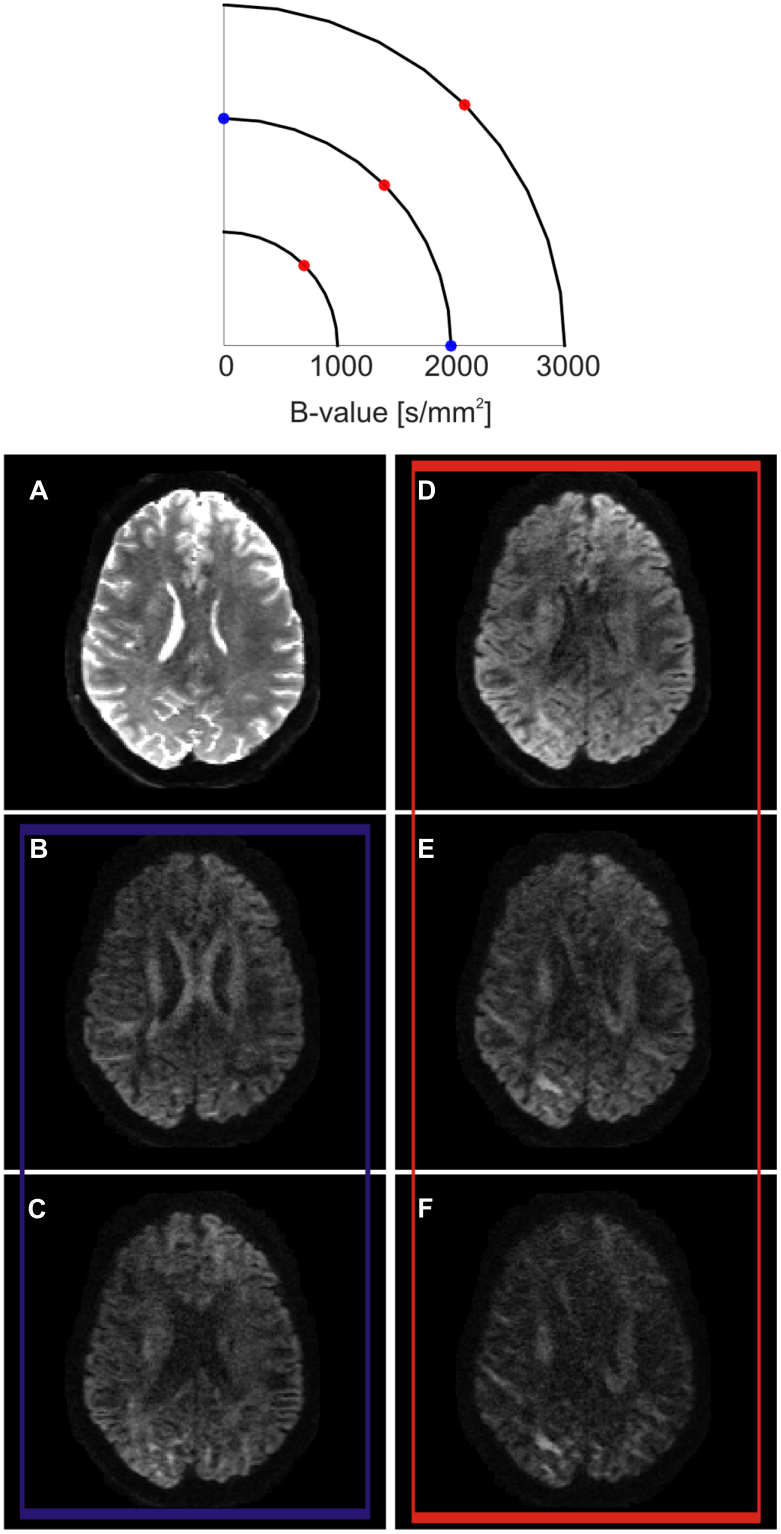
Q-space encoding example. Q-space is represented as a two dimensional space for simplification purposes (top). Three different shells at three different *b*-values are outlined. On each shell, several diffusion encoding directions (q-vectors) are sampled. Example axial slice obtained at different positions in q-space (bottom). (A) When the diffusion encoding gradients are switched off, the so-called T2-weighted b0 volume is obtained. (B,C) show the effect of sampling q-space at two different diffusion encoding orientations lying on the same b-shell. While SNR and amount of probed diffusion are kept constant, the contrast changes at different anatomical locations. When sampling along the left–right direction, the body of the corpus callosum show a higher signal drop (C) in respect to the sampling along the anterior-posterior direction (B). This reflects the fact that, in the body of the corpus callosum, axons are mainly oriented along the left–right orientation. (D–F) show the effects of sampling along the same diffusion encoding orientation while increasing *b*-value. In this case, the total SNR decreases while moving from lower to higher *b*-values, but the contrast increases. As an effect, different anatomical structures are more strongly differentiable between each other according to their main axonal orientation.
